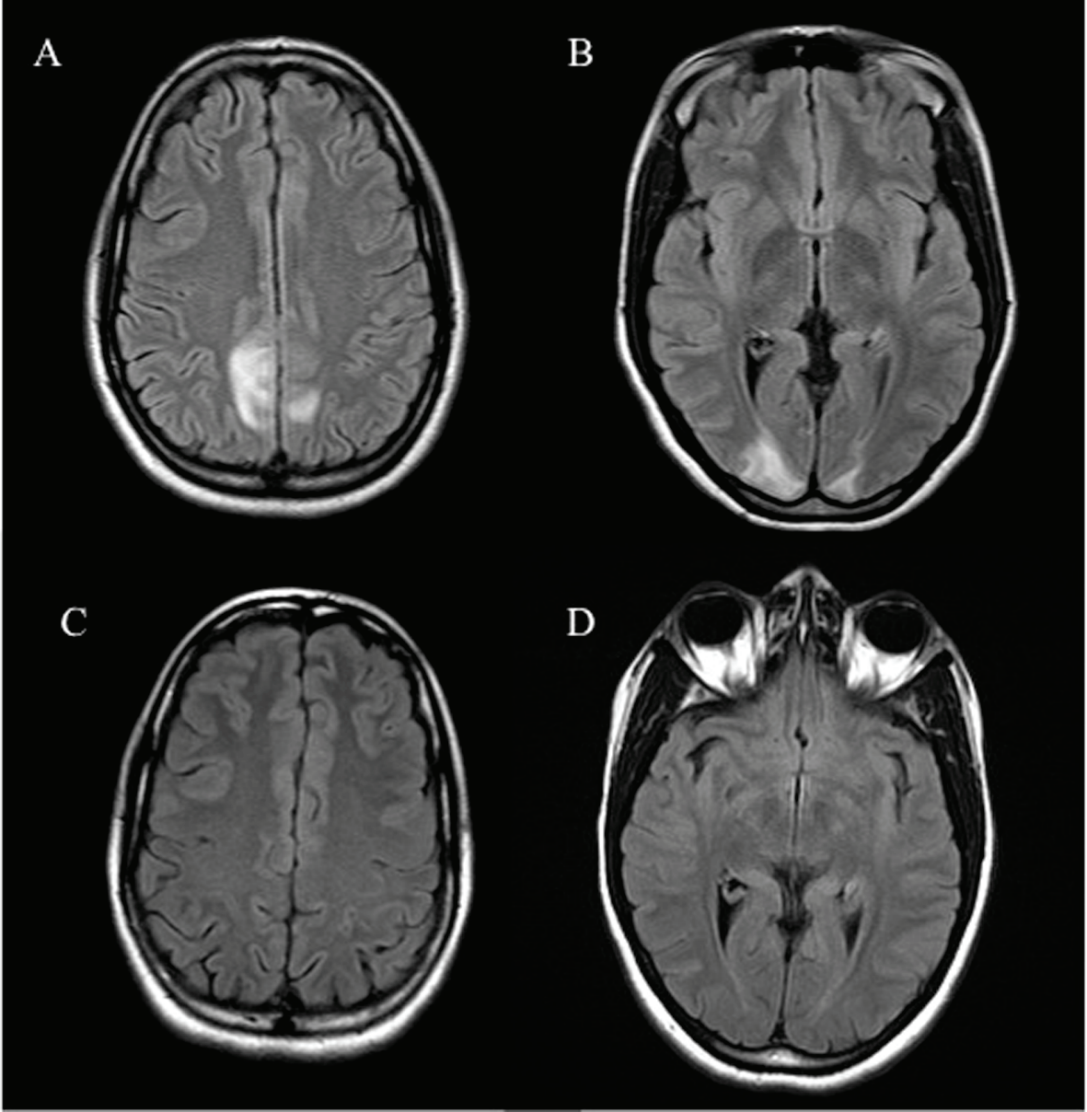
Axial FLAIR sequence MRI of the brain reveals hyperintensities in the parieto-occipital regions (A, B) which resolved on repeat MRI (C, D).
